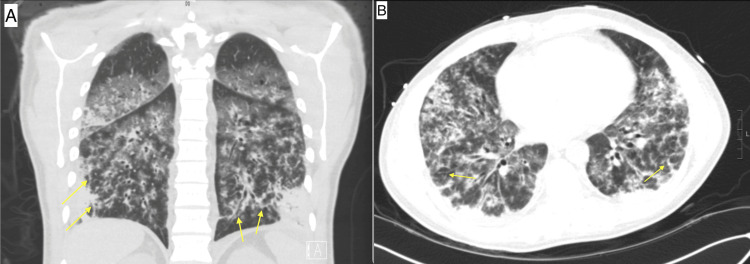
The CT scan of the chest shows multifocal consolidative and ground glass opacities with tree-in-bud nodularity (yellow arrows) in the coronal (A) and axial (B) views
